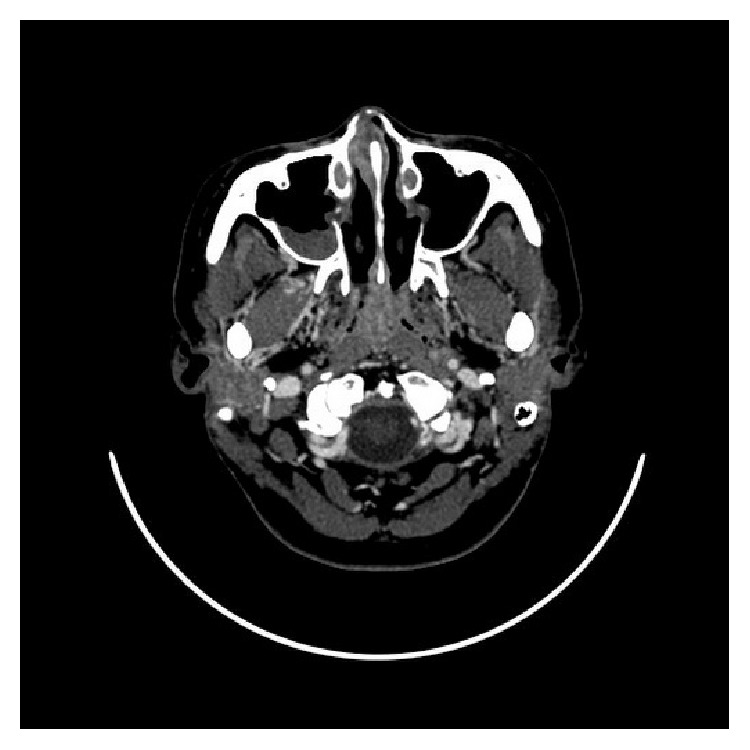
A 32-year-old woman, axial contrast-enhanced CT shows diffuse mucosal thickening without invasion into surrounding structures.
